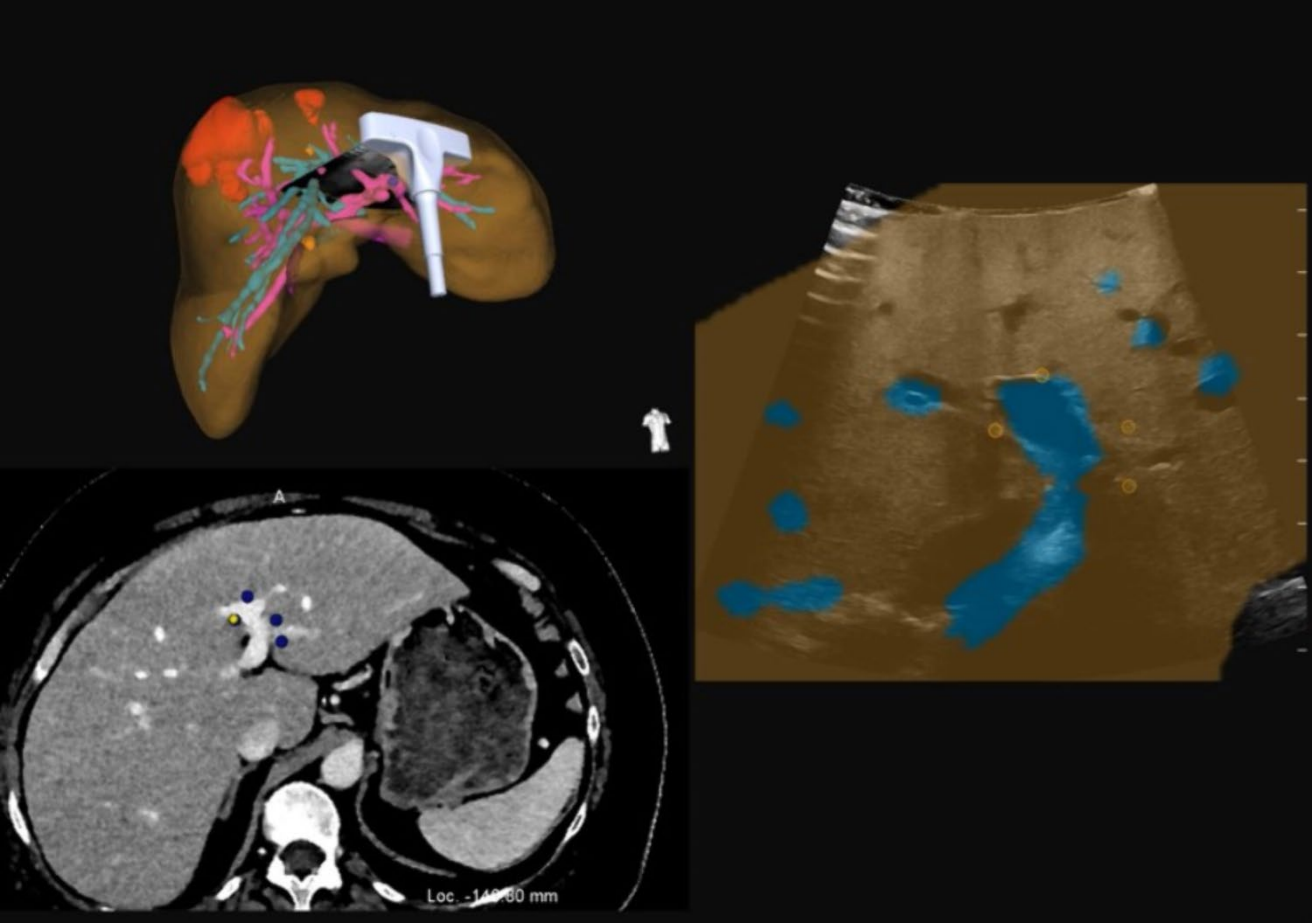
Screenshot of the intraoperative CAScination Display after the finished registration process via the LPV Right image: Ubersound Display superimposing the colored vir3DLiver onto the IOUS image in two-dimensional space. Set registration landmarks around the LPV are marked as orange circles. Left upper image: overlay of the IOUS and the vir3DLiver in three-dimensional space. Left lower image: Abdominal CT-Scan with 4 set registration landmarks around the LPV (blue/yellow dots)
